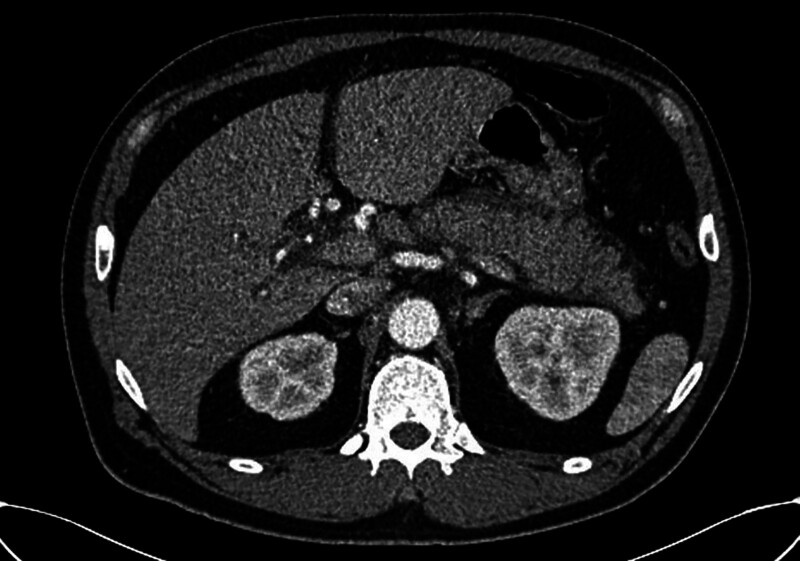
Abdominal CT showing edematous change in the left anterior segment of pancreas, suggestive of acute pancreatitis.
